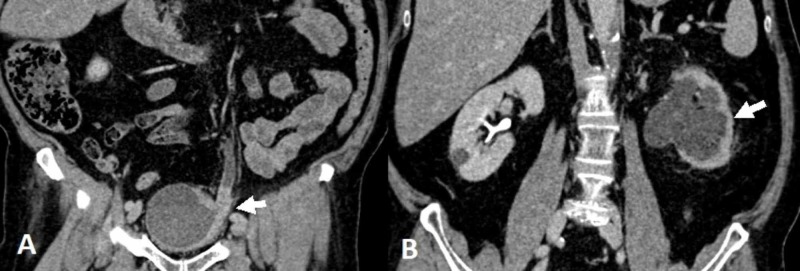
Enhanced computed tomography scan (A) Coronal view showing urothelial thickening at the level of ureteral re-implantation site (arrow), also noted is a left lateral bladder wall thickening; (B) Another coronal view of the upper tracts revealing a hydronephrotic left kidney, with effaced parenchyma and decreased uptake of contrast (arrow).
